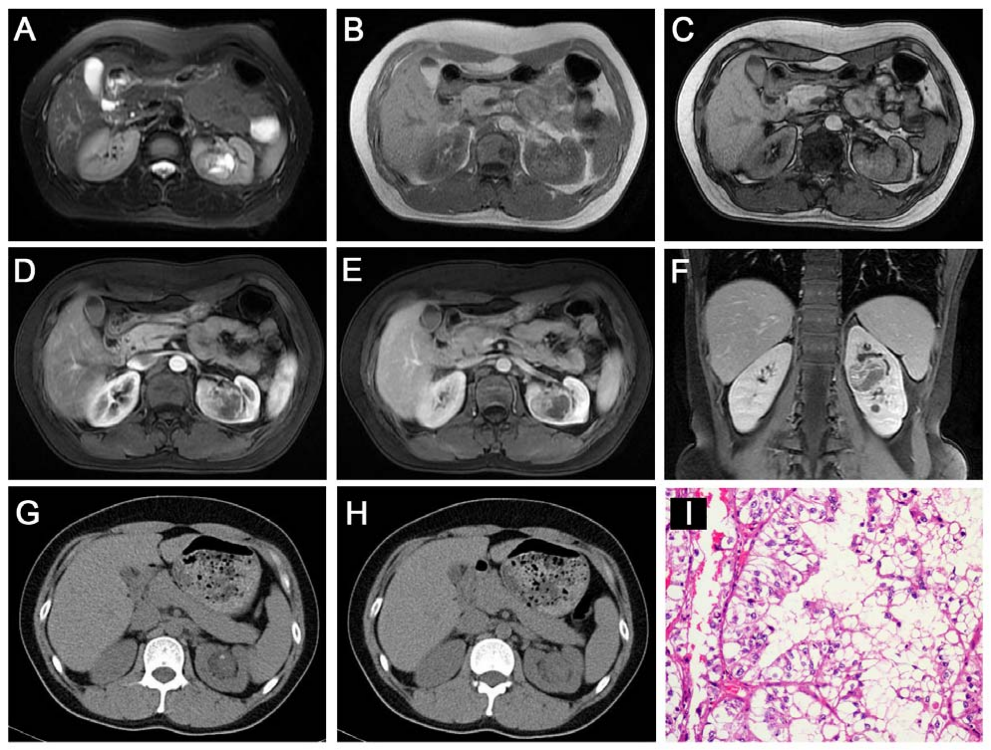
Translocation RCC in a 36-year-old woman (patient 9 in Table 1, 2, 3) with gross hematuria. A–C, Axial T2WI and plain (non-contrast) T1WI (B, IP in phase; C, OP) showing an irregular well-defined mass (T2, iso-high mixed signal intensity; T1, slightly high signal intensity) with a patchy area of cystic necrosis in the left renal pelvis. Axial CMP (D) and NP (E) gradient-echo MR images showing moderate heterogeneous enhancement in the left renal mass. F, Coronal delay phase image showing a delayed-enhancement mass within the contour of the kidney. G–H, Unenhanced CT images showing a well-defined, slightly high-density renal mass with mottling calcification. I, The tumor cells contain hyaline cytoplasm with a papillary structure (HE 10 & 40).
